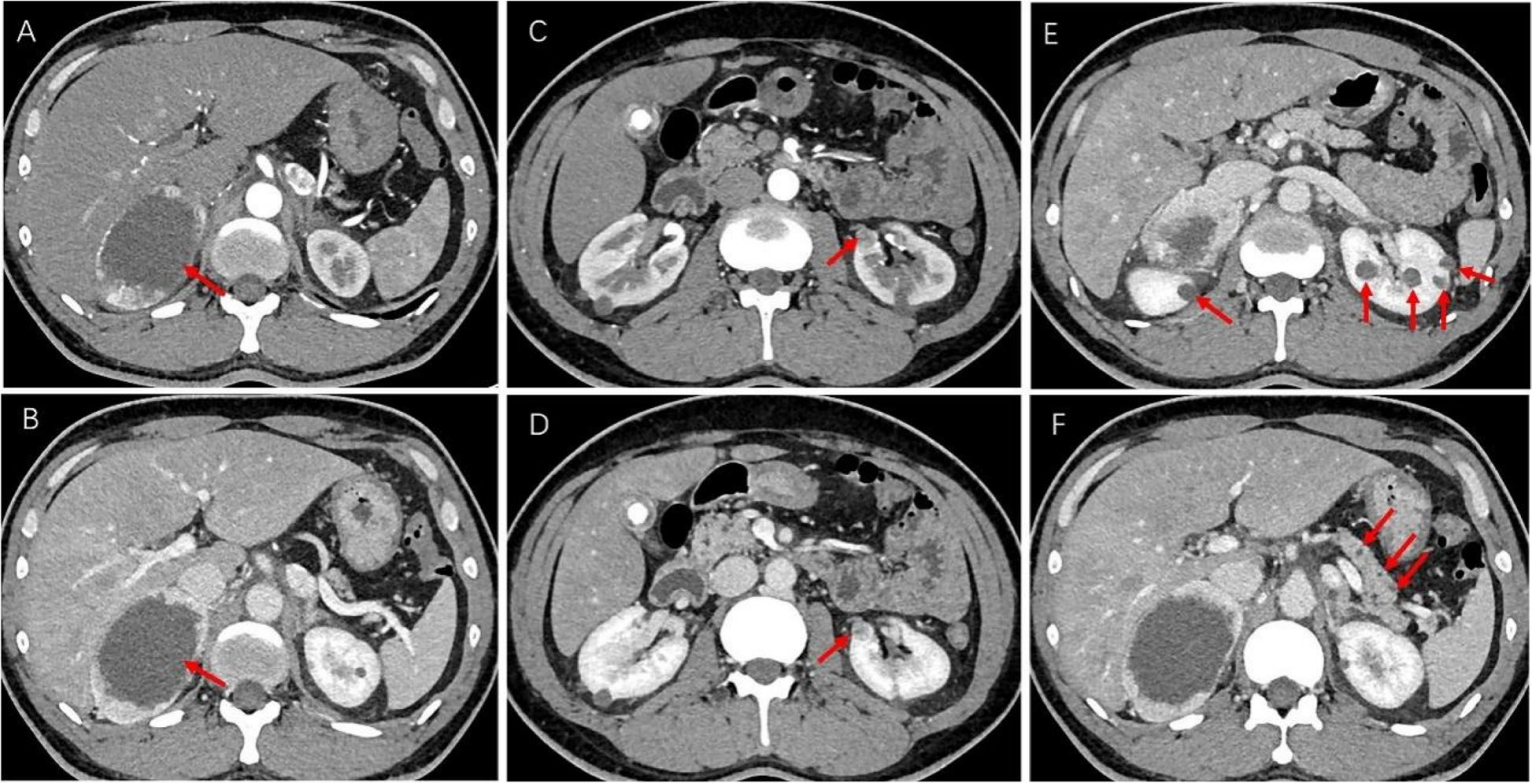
Contrast enhanced abdominal CT. A, B Pheochromocytoma (arrow) of the right adrenal gland. A Arterial phase. B Venous phase. C, D Renal carcinoma (arrow) of the left kidney. C Arterial phase. D Venous phase. E Multiple cysts in both kidneys (arrow). F Multiple cysts in pancreas (arrow)
